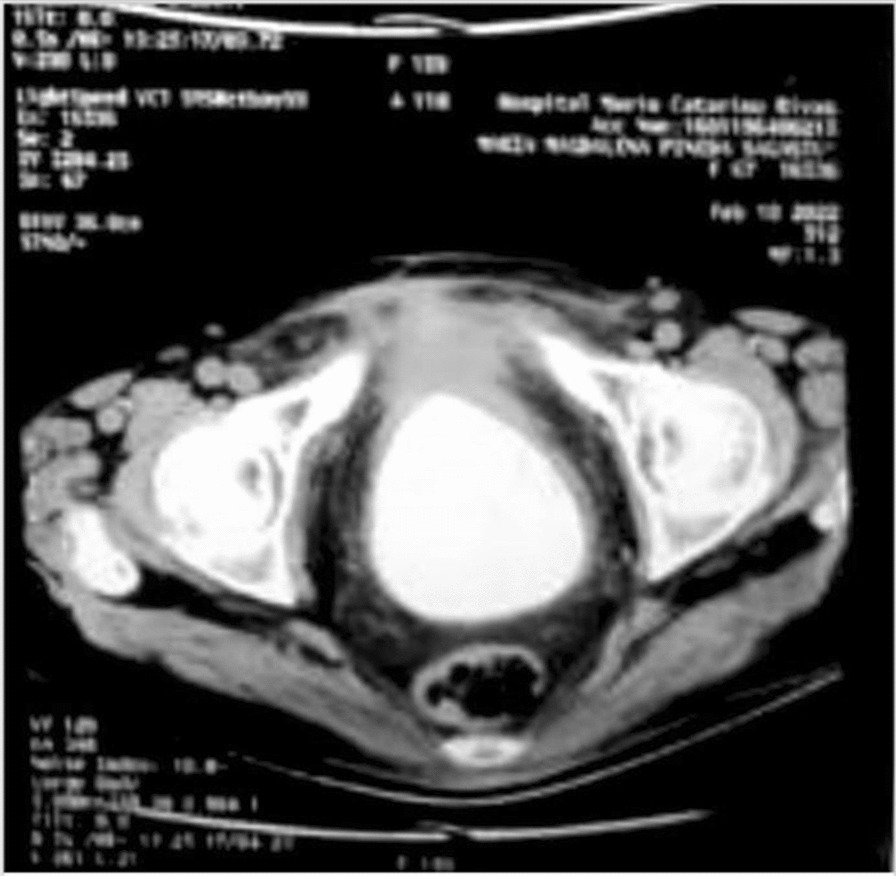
Plain computed tomography scan at the pelvic level, depicting a hyperdense ovoid mass located within the urinary bladder, consistent with a bladder stone
